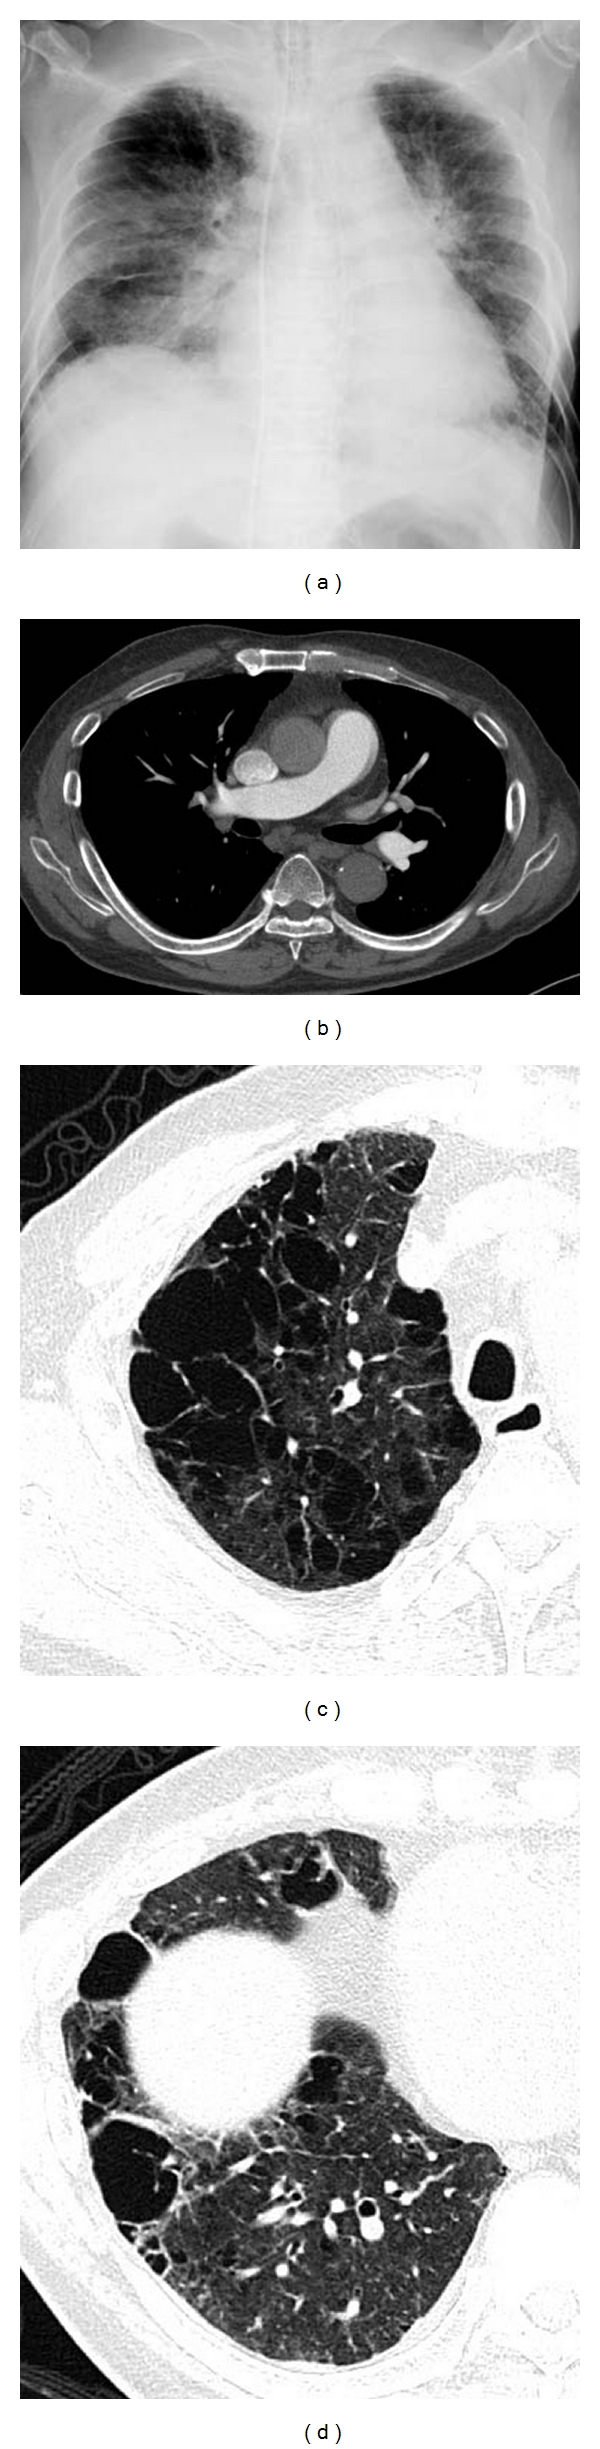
Emphysema with fibrosis, pulmonary hypertension. Estimated right ventricular pressure by cardiac ultrasound is 80 mmHg. (a) Chest X-ray shows cardiomegaly with enlargement of central pulmonary arteries. (b) Contrast-enhanced CT shows dilatation of central pulmonary arteries. There is no evidence of pulmonary thromboembolism. (c) High-resolution computed tomography (HRCT) of upper lung shows severe pulmonary emphysema; some cystic lesions have thick wall. (d) HRCT of the lower lung shows marked destructive change in the lung. There are several large cysts with thick wall.
